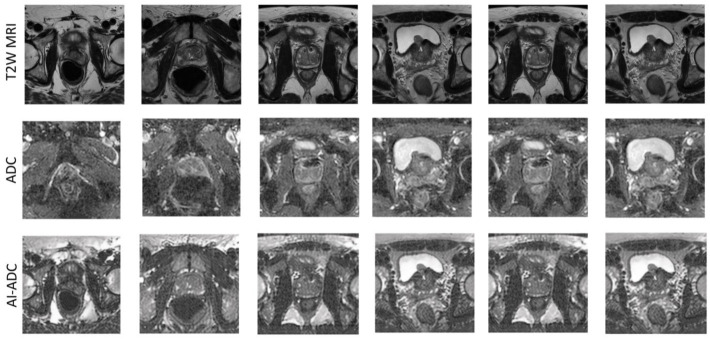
Failure analysis in unsegmented MRI approach. Initially, we utilized full-size T2W MRIs and ADC maps, resulting in failure due to variability in prostate size. Contrasting with our initial method, the current approach employs cropped images guided by the prostate segmentations.
